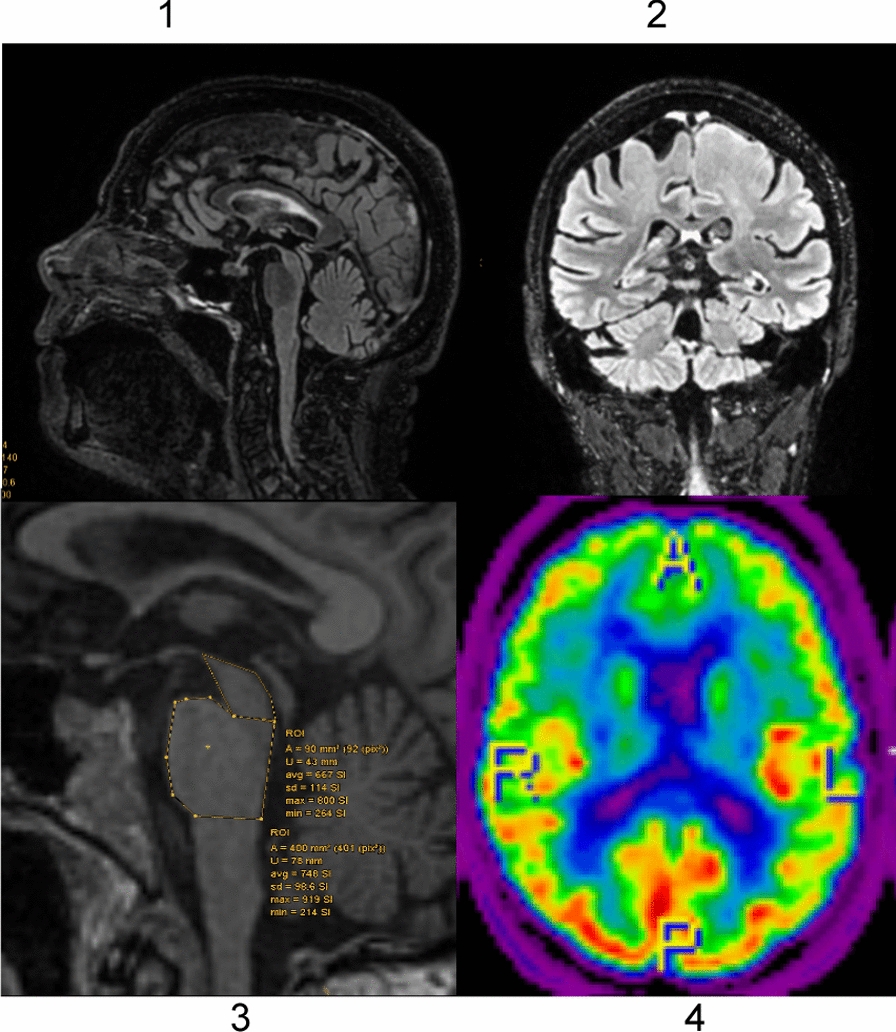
1–4. 1–3: FLAIR MRI, 4: FDG-PET. 1: sagittal, hummingbird sign. 2: coronar, atrophy of superior cerebellar peduncle 3: sagittal, pons-midbrain ratio of 0.225.4: axial, high metabolism occipital, low metabolism frontal and in caudate nucleus
